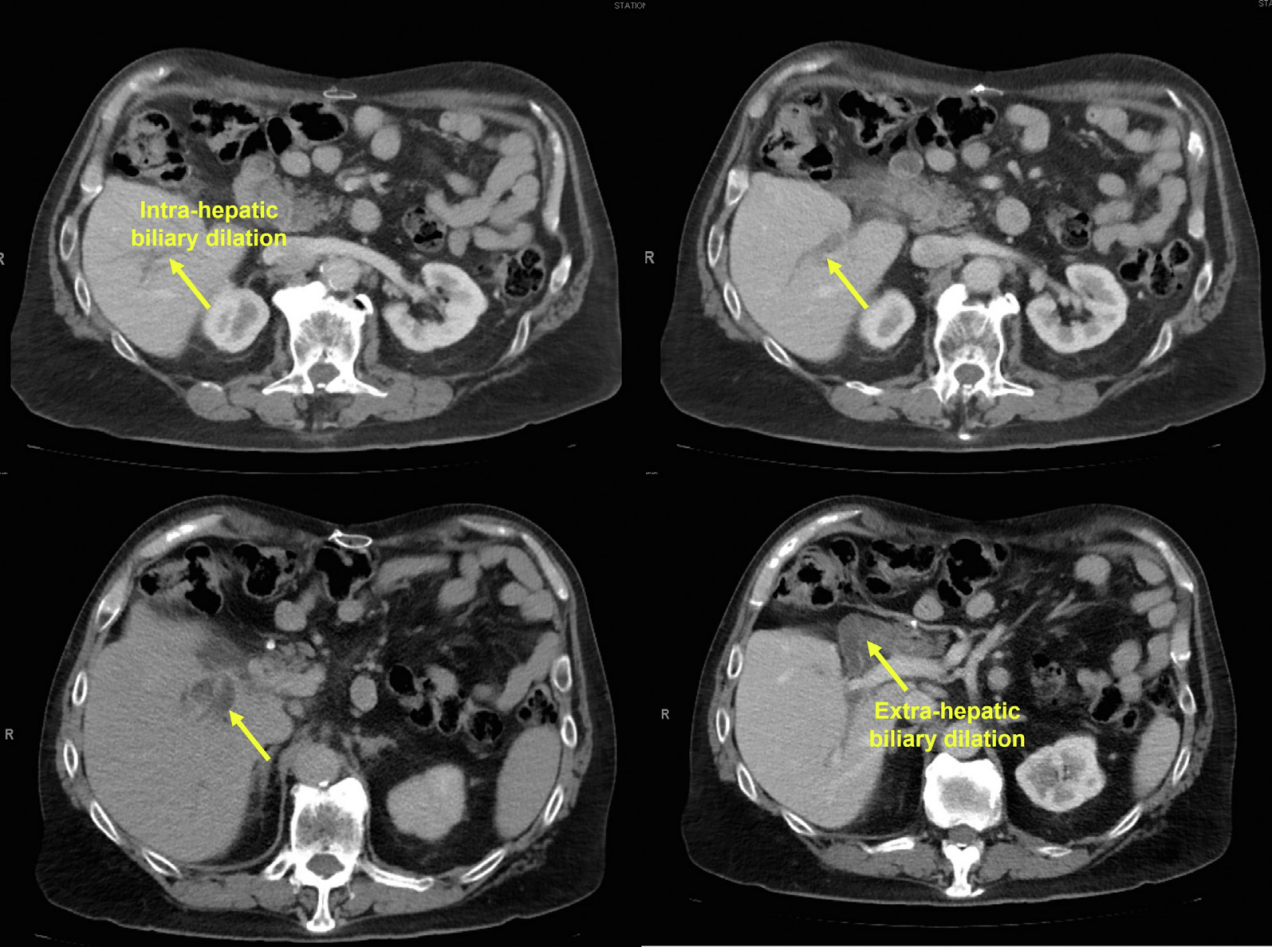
Transverse plane liver CT scan indicating dilation of the intrahepatic and extrahepatic bile ducts.
